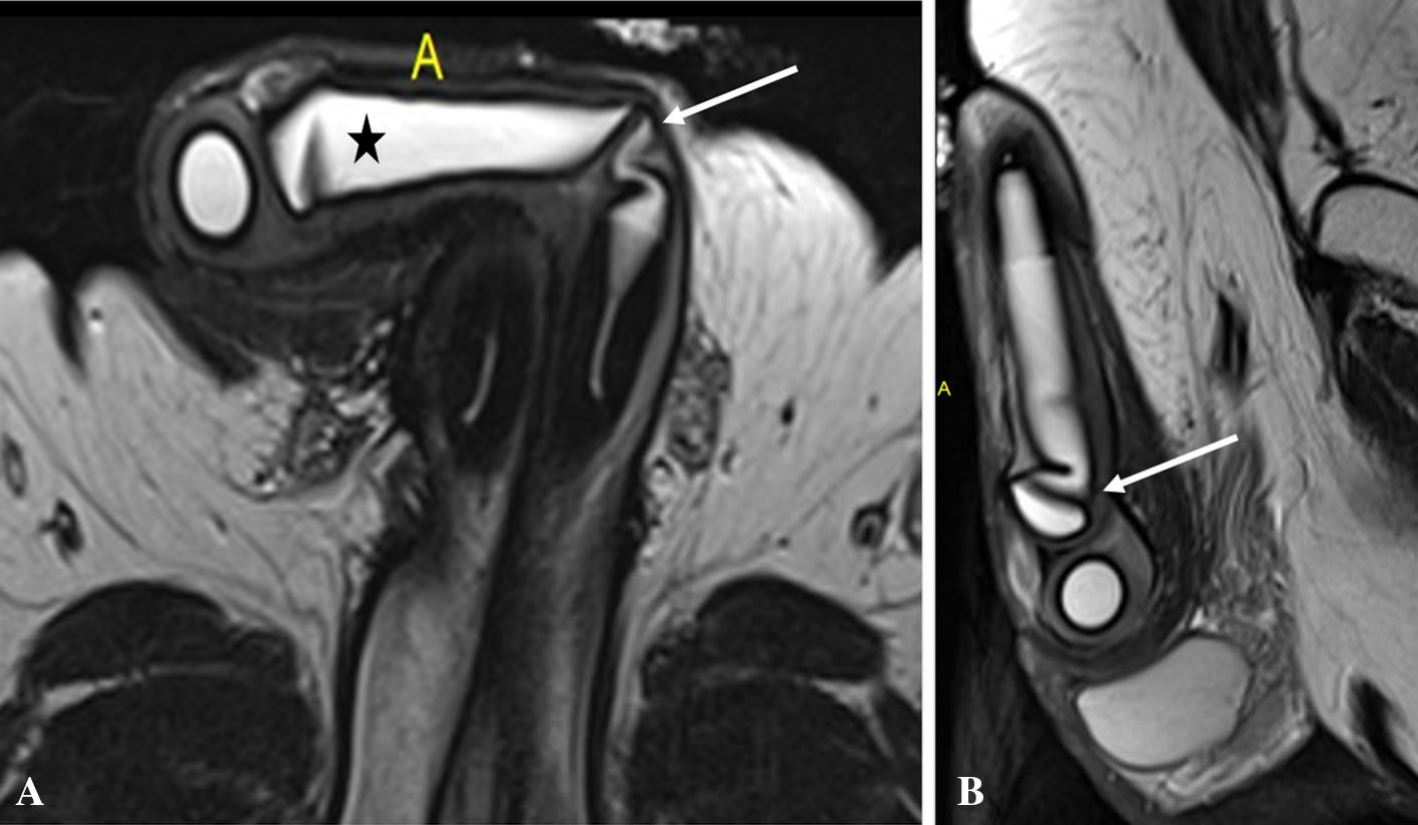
70-year-old man who had penile prosthesis insertion 6 years back presented with poor erection. Axial (a) and sagittal (b) T2-weighted MRI shows the buckling deformity (arrows) in the posterior part of one of the cylinders (asterisk)
